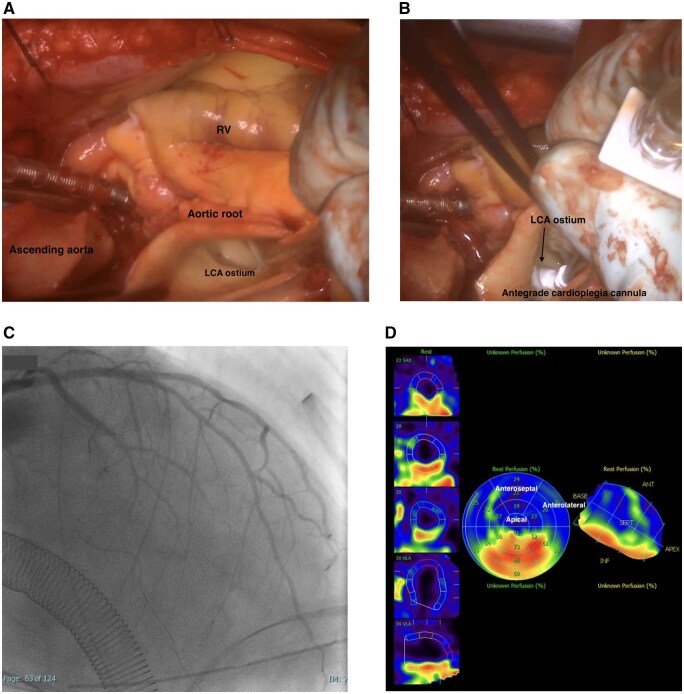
Intraoperative and Postoperative Assessment. (A) Intact left coronary ostium. (B) Selective antegrade cardioplegia into the left coronary ostium; the left coronary ostium and the antegrade cardioplegia cannula are labelled. (C) Postoperative coronary angiography showing patent coronary arteries. (D) Resting perfusion single-photon emission computed tomography showing extensive fixed defects in the left coronary distribution.
